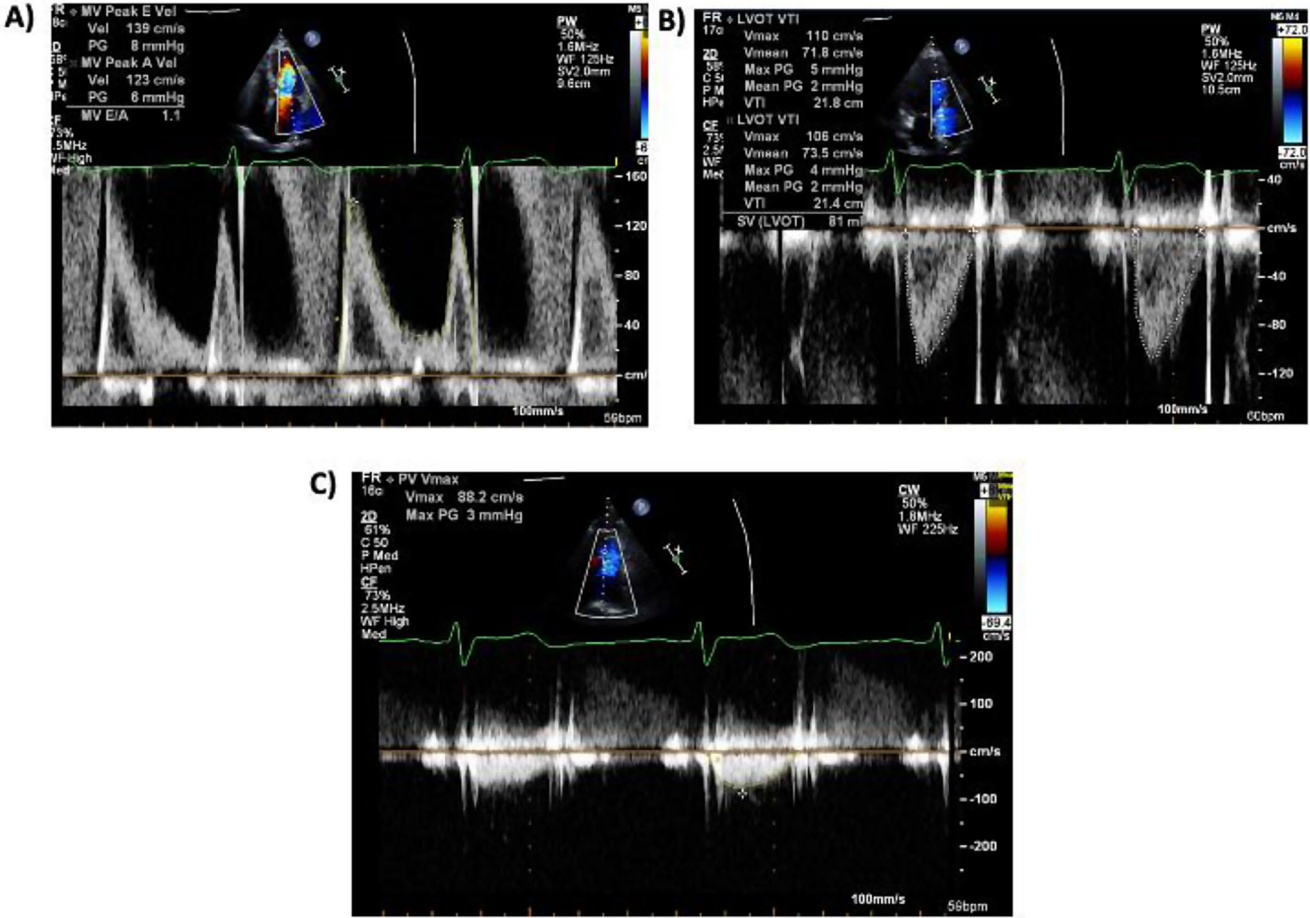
Example of the measurement of the mitral valve VTI, LVOT VTI and RVOT Continuous Wave Doppler VTI in a patient with severe mitral regurgitation. Panel A (top left) shows the calculation of the MV VTI which is performed by pulse wave Doppler at the leaflet tips in the apical 4 chamber view (trace in yellow outline). Panel B (top right) shows the calculation of the LVOT VTI performed by pulse wave Doppler of the LVOT in the apical 3 chamber view (trace in white outline). The is also measured in the apical 5 chamber view and the results averaged. Panel C (bottom) shows the calculation of the RVOT VTI which is performed by continuous wave Doppler of the RVOT in the parasternal short axis view (trace in yellow outline). The MV VTI: LVOT VTI Ratio is 41/21.6 = 1.9. The MV VTI: RVOT VTI Ratio is 41/17.4 = 2.4
